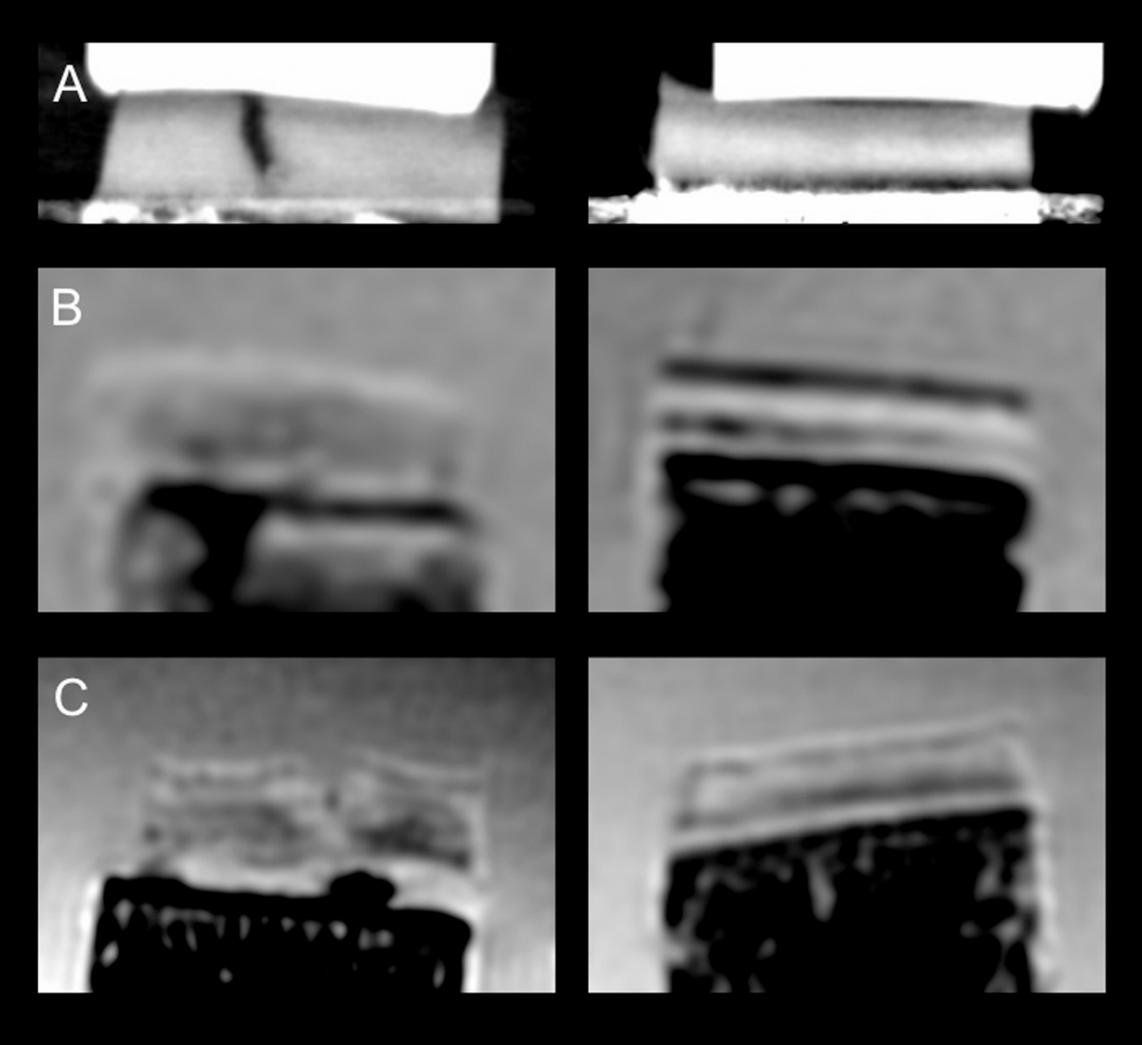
Morphological images of one osteochondral sample with a morphological cartilage defect (left column) and one intact osteochondral sample (right column) for all different imaging modalities. A: PCCT. B: 3T MRI, IM-w fs sequence. C: 7T MRI, IM-w fs sequence. The defect is clearly depicted on the PCCT image, while it may only be suggested on MR images.
